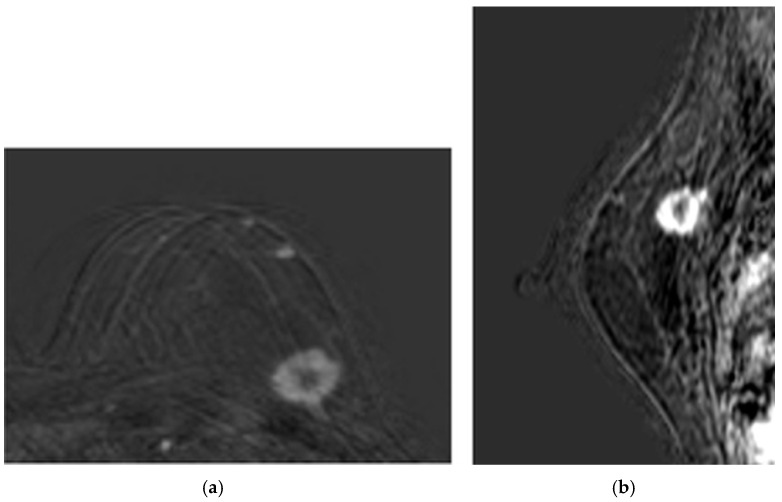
Baseline CE-MRI of Patient 1: (a) sample axial projection; (b) sample sagittal projection. The lighter areas, on the gray/black background, depict the tumoral ROIs.
